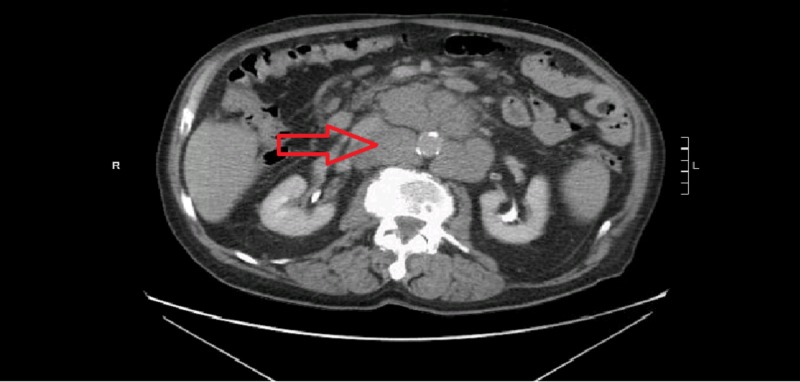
Computed tomography (CT) scan abdomen. Extensive paraaortic lymphadenopathy (red arrow).
